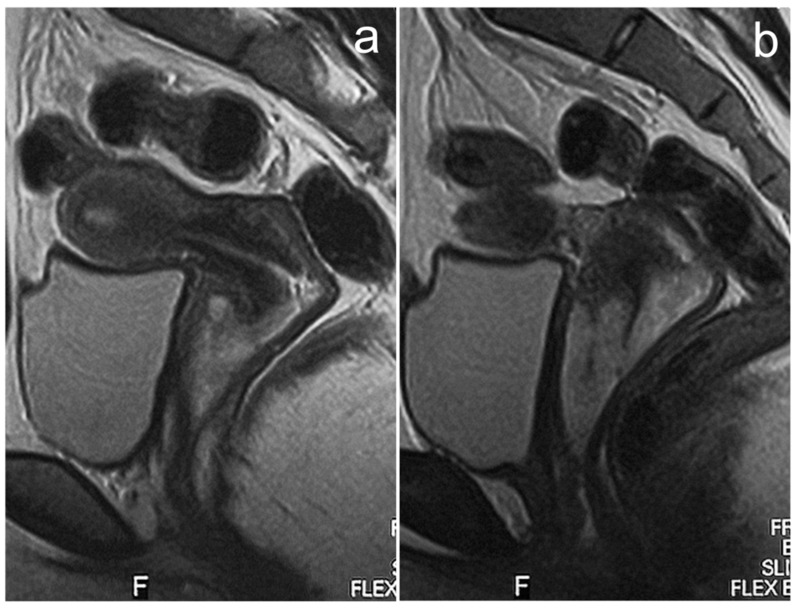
MRI of cervical cancer in 16-year-old girl (a,b). (a,b)—FSE/T2.
